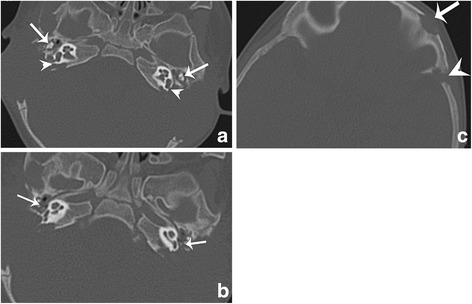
Axial HRCT of the temporal bone demonstrates diffuse and symmetric destruction of the bilateral temporal bone. The ossicular chains remain intact (arrow in a). Both of the posterior SCCs are completely destructed (arrowhead). The smallest bone stapes are still in good condition even if they are surrounded by LCH tissue (arrow in b). Axial CT scan also shows the erosion of the frontal bone and squama (c)
